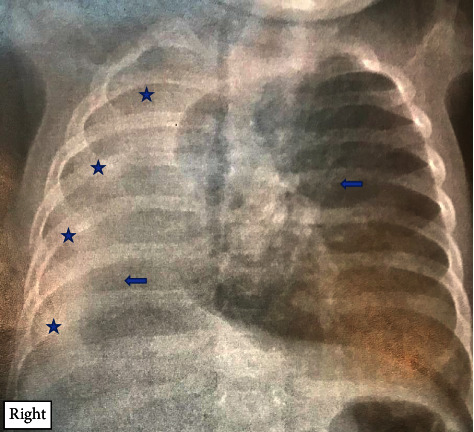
Frontal chest radiograph in a 4-month-old infant, showing right thoracic opacity (stars), hypertrophy and mediastinal herniation of the left lung (arrows), and lack of visualization of the left stem bronchus.
